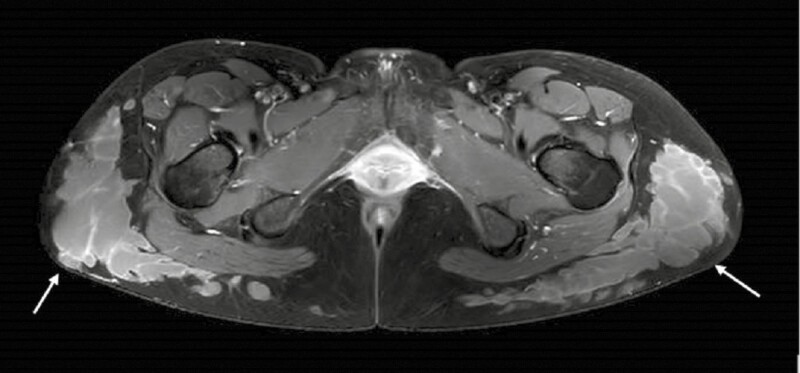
MRI scan of the gluteal region (transversal view): accumulation of poorly defined fillers in different fat layers of both buttocks (white arrows) extending from the gluteal groove to the anterolateral area of the thigh.
